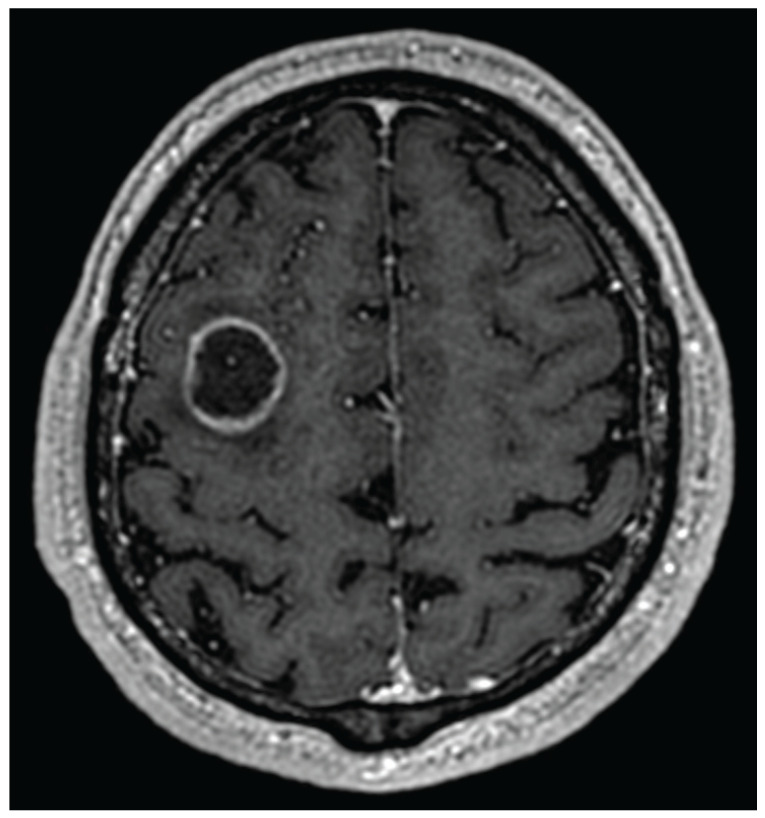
Glioblastoma with ring enhancement on MRI T1 with contrast.
